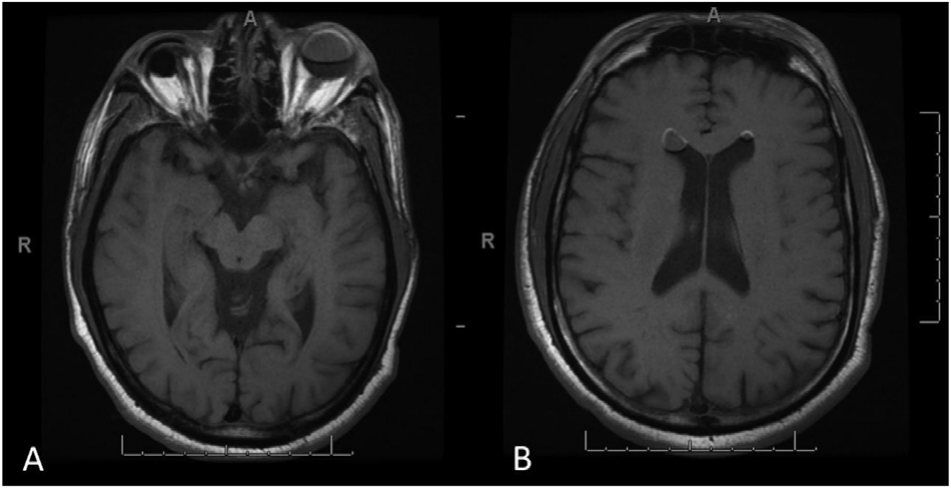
(A) Axial T1-weighted MRI at the level of the eyes showing again the previously performed right eye evisceration and acrylic conformer insertion, and an ovoid-shaped isointense signal in the left globe that corresponds to intraocular silicone oil and has the same signal signature with fat. (B) Axial T1-weighted MRI at the level of the lateral ventricles showing 2 ovoid hyperdensities in the frontal horns bilaterally.
